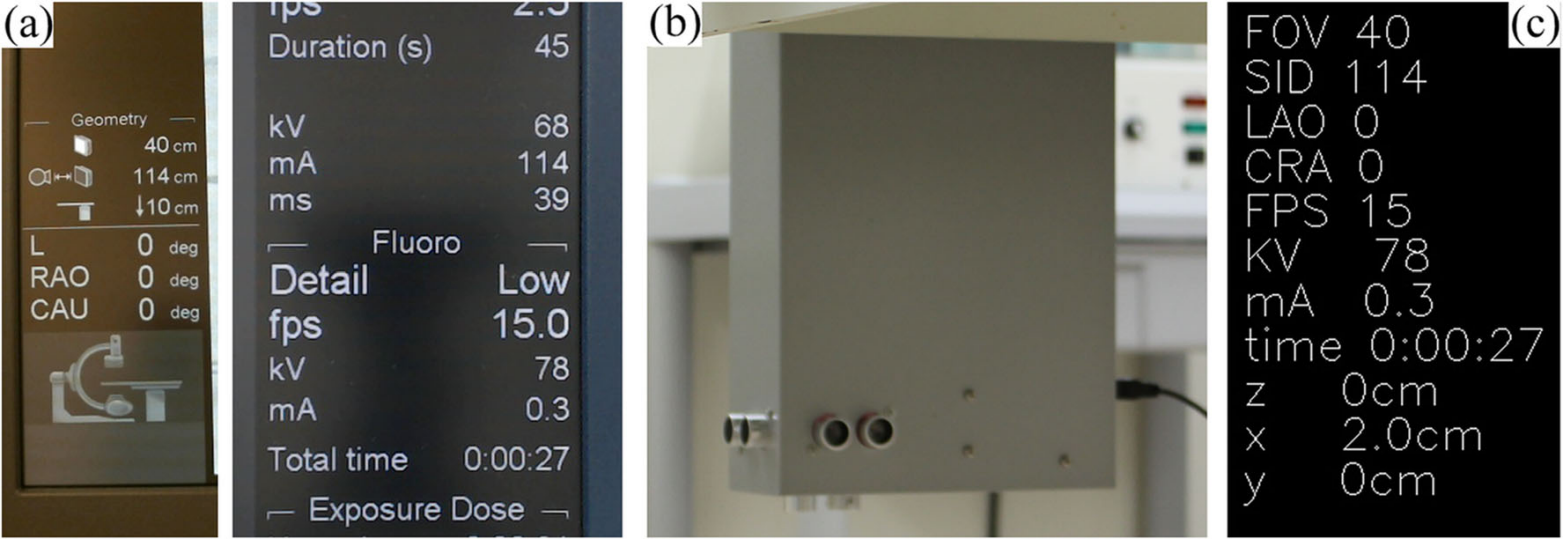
a Captured screen images from Innova 4100-IQ using web cameras for obtaining fluoroscopic conditions. b The ultrasonic sensor unit comprises three ultrasonic sensors facing three directions for measuring the table position. This sensor unit was installed under the table and was connected directly to the host server. c Recognized fluoroscopic conditions necessary for FDEIR from captured images and the sensor unit
